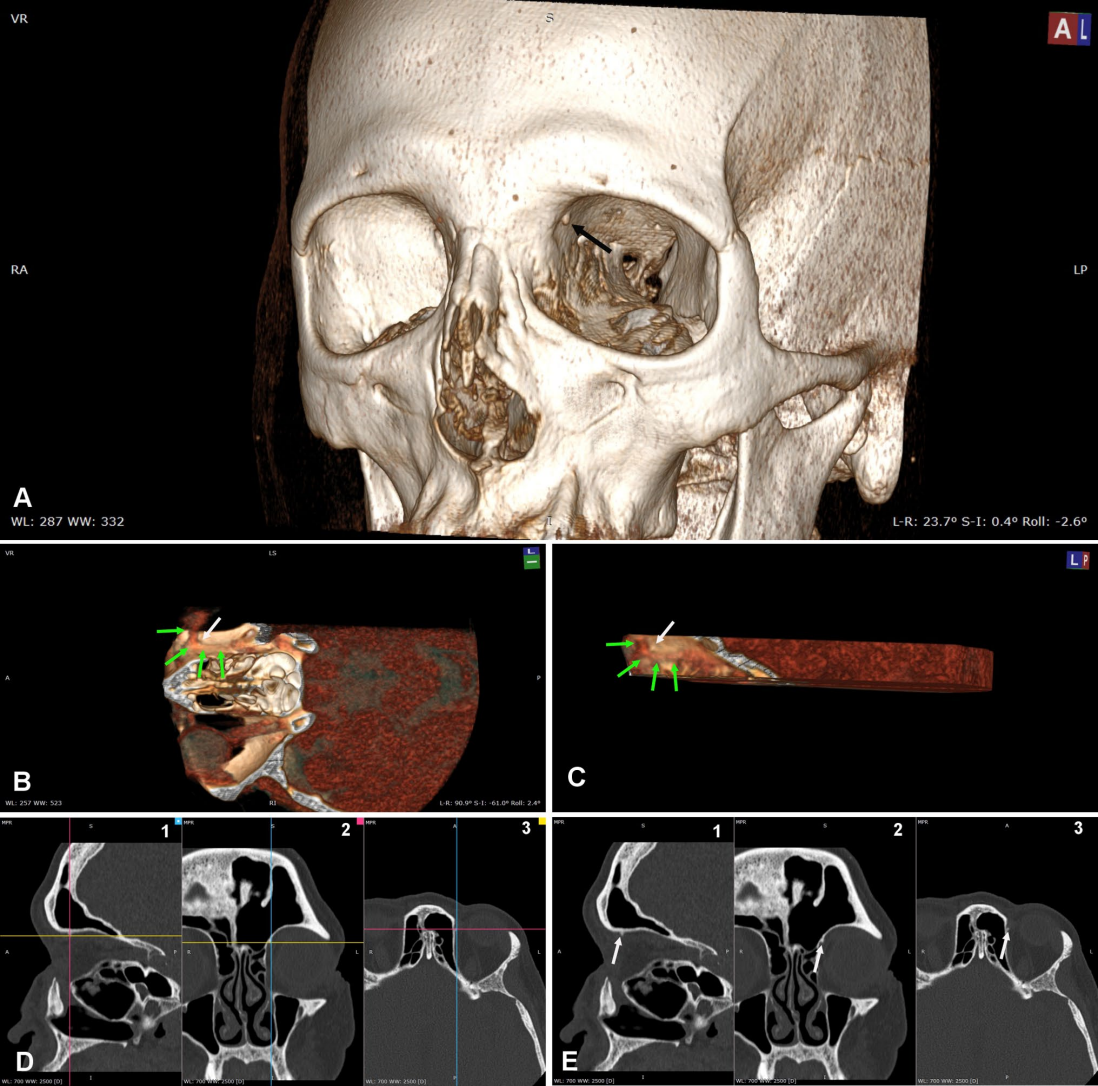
CT of a left orbit with a trochlear spine. A A prominent trochlear spine can be seen (arrow). B, C The adjustment of the image window allows to visualize intraorbital structures. To observe the superior oblique and its tendon (green arrows), lower and upper planes were clipped away as well as the lateral part of the orbit and the eye. B is an inferior view slightly tilted to the left. The superior oblique (green arrows) can be seen passing medial to the trochlear spine (white arrow) before bending to run towards the eye. In C the same object has been rotated to be seen from the left and slightly from behind. The superior oblique (green arrows) can be seen passing under the trochlear spine (white arrow). D, E The same trochlear spine can be seen in MRP. D demonstrate the orientation of the planes intersecting the spine. D1 shows the orientation of the frontal (magenta) and axial (yellow) planes as seen on the sagittal plane; D2 shows the orientation of the sagittal (blue) and axial (yellow) planes as seen on the frontal plane; D3 shows the orientation of the frontal (magenta) and sagittal (blue) planes on the axial plane. E is the same image seen in D without showing the planes to better visualize the spine (white arrows) on the sagittal (E1), the frontal (E2) and the axial (E3) planes
